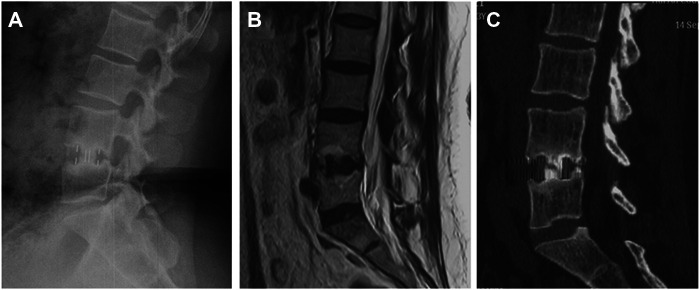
Case example of fusion after Grade I subsidence with no symptoms. 5 years of radiographs (A), MRI (B), and CT images (C) showing fusion obtained after placement of a standard cage despite subsidence occurrence.
